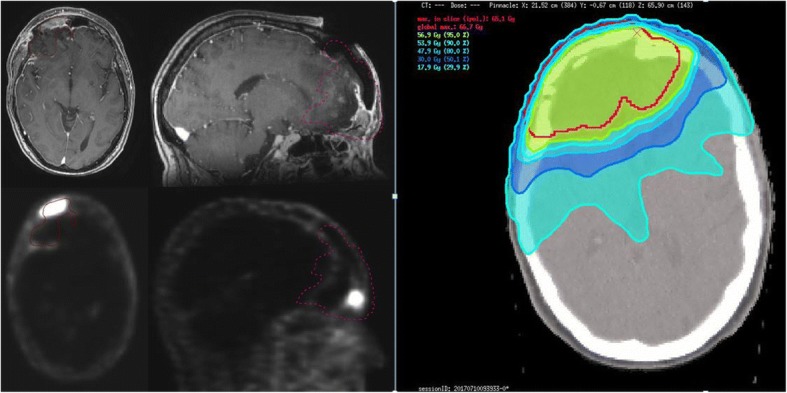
Example of a patient with a right frontal meningioma s/p subtotal resection while postoperative MRI does not unequivocally show a tumor residual but radiologists describe postoperative granulation tissue. The PET/CT scan nicely visualizes the bone involvement, on the right the IMRT plan including PTV and 95%/90%/80%/50%/30%-isodoses, respectively
